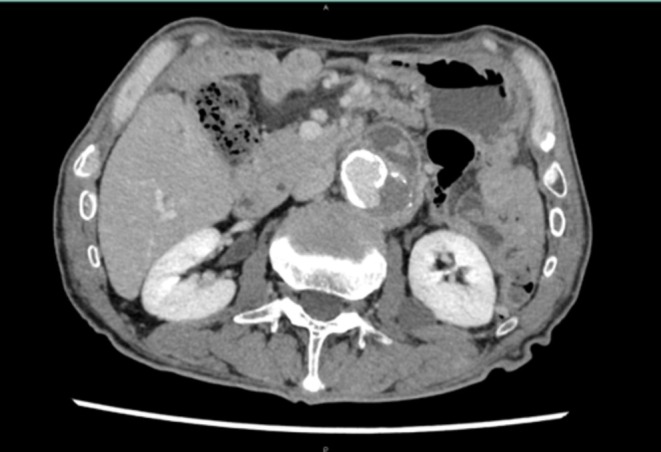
Contrast‐enhanced computed tomography at the time of aneurysmal rupture. Progressive enlargement of the abdominal aortic aneurysm and extensive spread of the surrounding abscess are observed.
